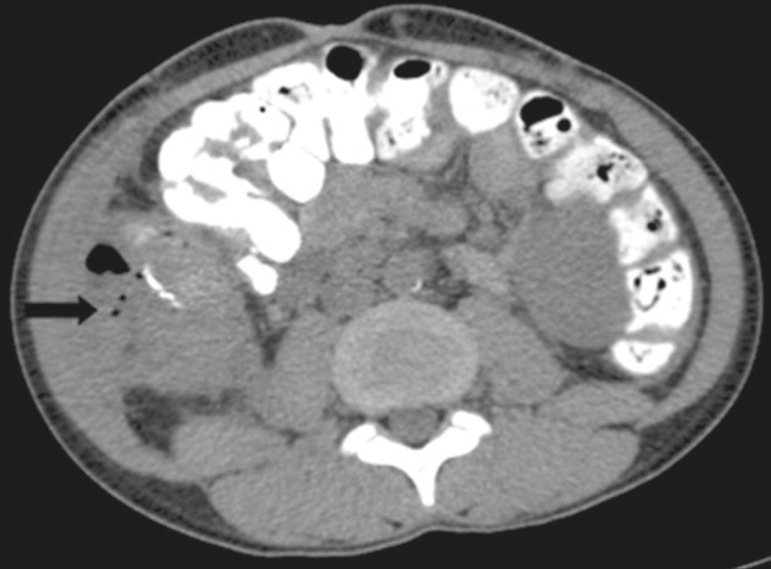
Axial CT with oral contrast of a patient in the early postoperative period of SPKT, with dehiscence of the anastomosis between the duodenal stump of the donor and the small intestine of the recipient, showing a fluid collection with gaseous foci adjacent to the topography anastomosis (arrow).
